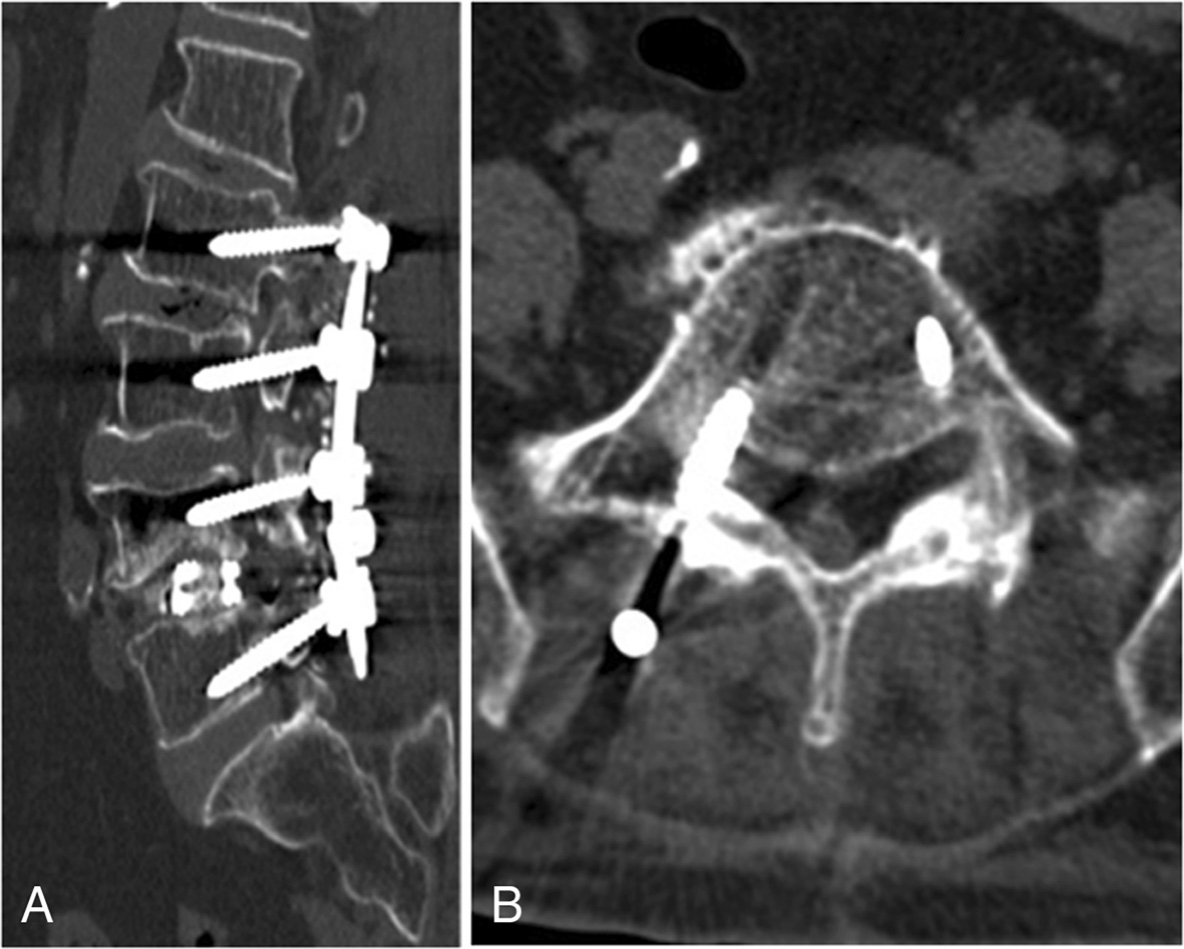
Computed tomography images of nerve root irritation. This patient had persistent pain in the right thigh and calf after L2–5 laminectomy, transpedicular screw fixation, and interbody fusion. a Sagittal and b cross-sectional postoperative computed tomography showed that the right L5 screw had been misplaced and cut out of the pedicle, causing right L5 nerve root irritation
